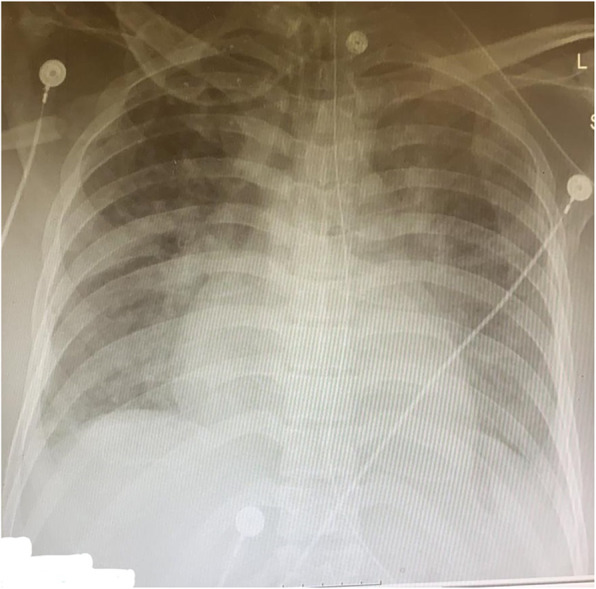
Chest x-ray at discharge from the ARDS specialized department, showing no infiltrates or consolidation
